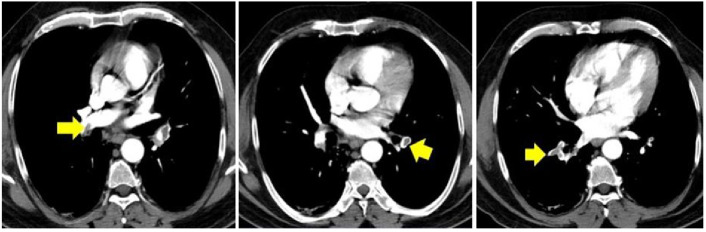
a-b-c: Chest CT of the patient who applied with diffuse chest pain and low oxygen saturation at 8weeks post-COVID: bilateral pulmonary embolism. (From archives of Aydın Yılmaz.
